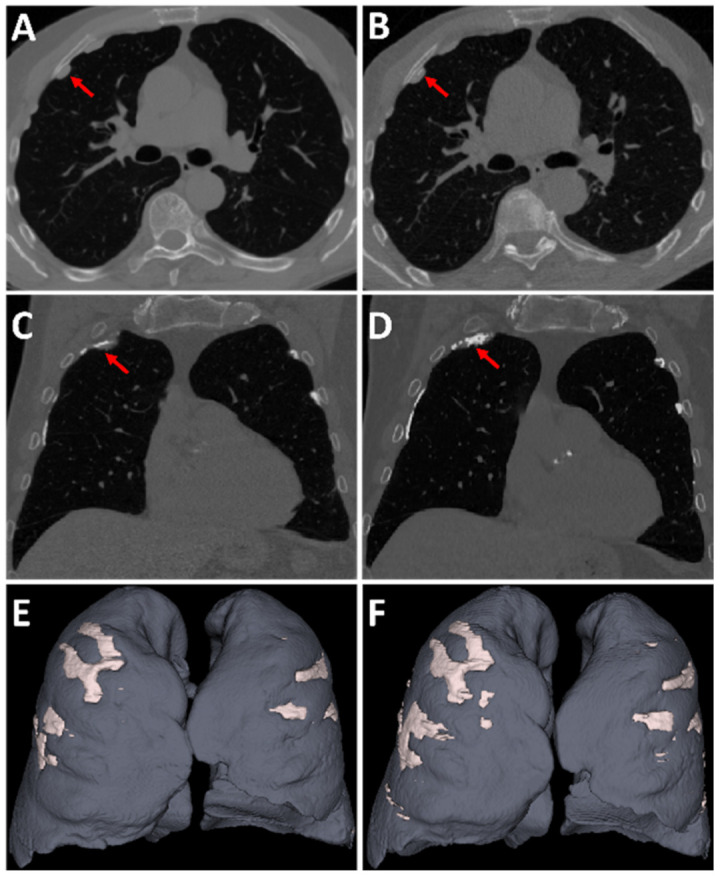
CT images of 71 years-old male at the 2nd (left panel) and the 3rd (right panel) CT screening rounds. Note the increase in pleural plaques volume ((E) 28.01 mL and (F) 49.25 mL), with the increase in calcifications (red arrows). (A,B) Axial native CT images (1 mm slice thickness); (C,D) Coronal native CT images; (E,F) 3D-volume rendering of CT images.
